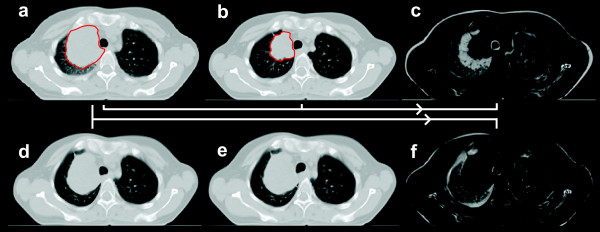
Patient # 6: a) planning CT; b) follow-up CT at the end of treatment; c) difference image between planning CT and follow-up CT; d) deformed follow-up CT using all target and organs-at risk meshes; e) deformed follow-up CT using target, lung and spinal cord; f) difference image between planning CT and deformed follow-up CT based on all target organs-at risk meshes. The contours of the macroscopic primary tumor in the planning CT and the follow-up CT are shown in red. Note the distortion of the vertebral body and the trachea without using meshes of these organs for deformable registration in e).
